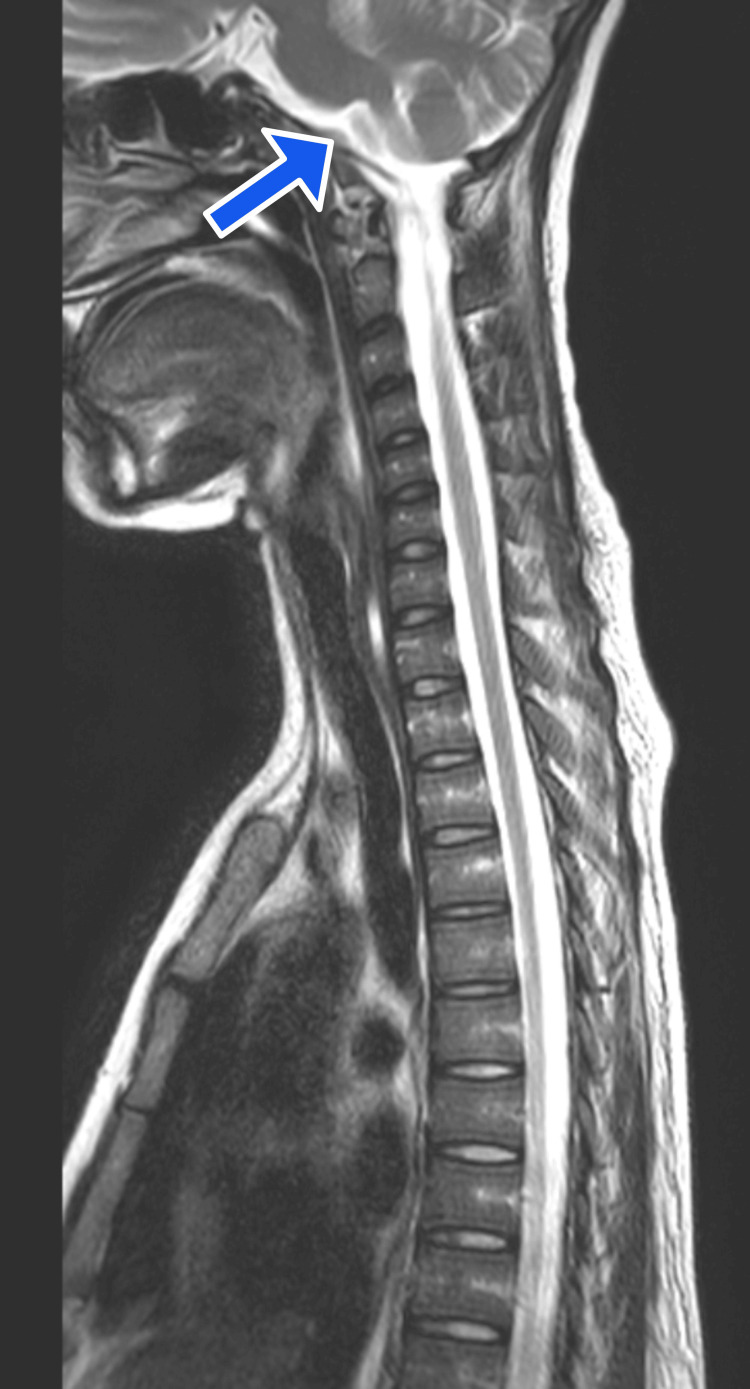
MRI brainstem and spine with a blue arrow showing high signals in the rostral medulla
